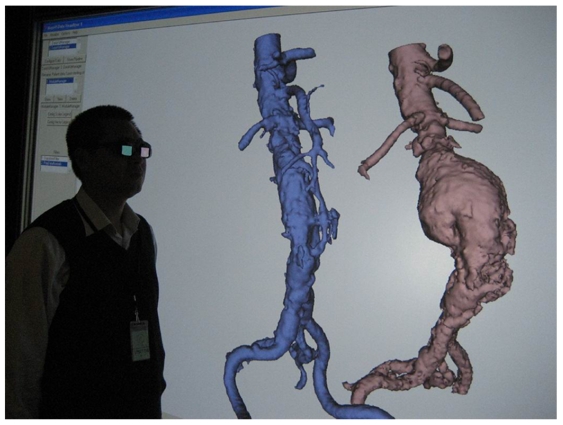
Stereo glasses are used to view CT volume data in a stereoscopic projection. Specialised hardware is required for stereoscopic viewing with the stereo glasses.
